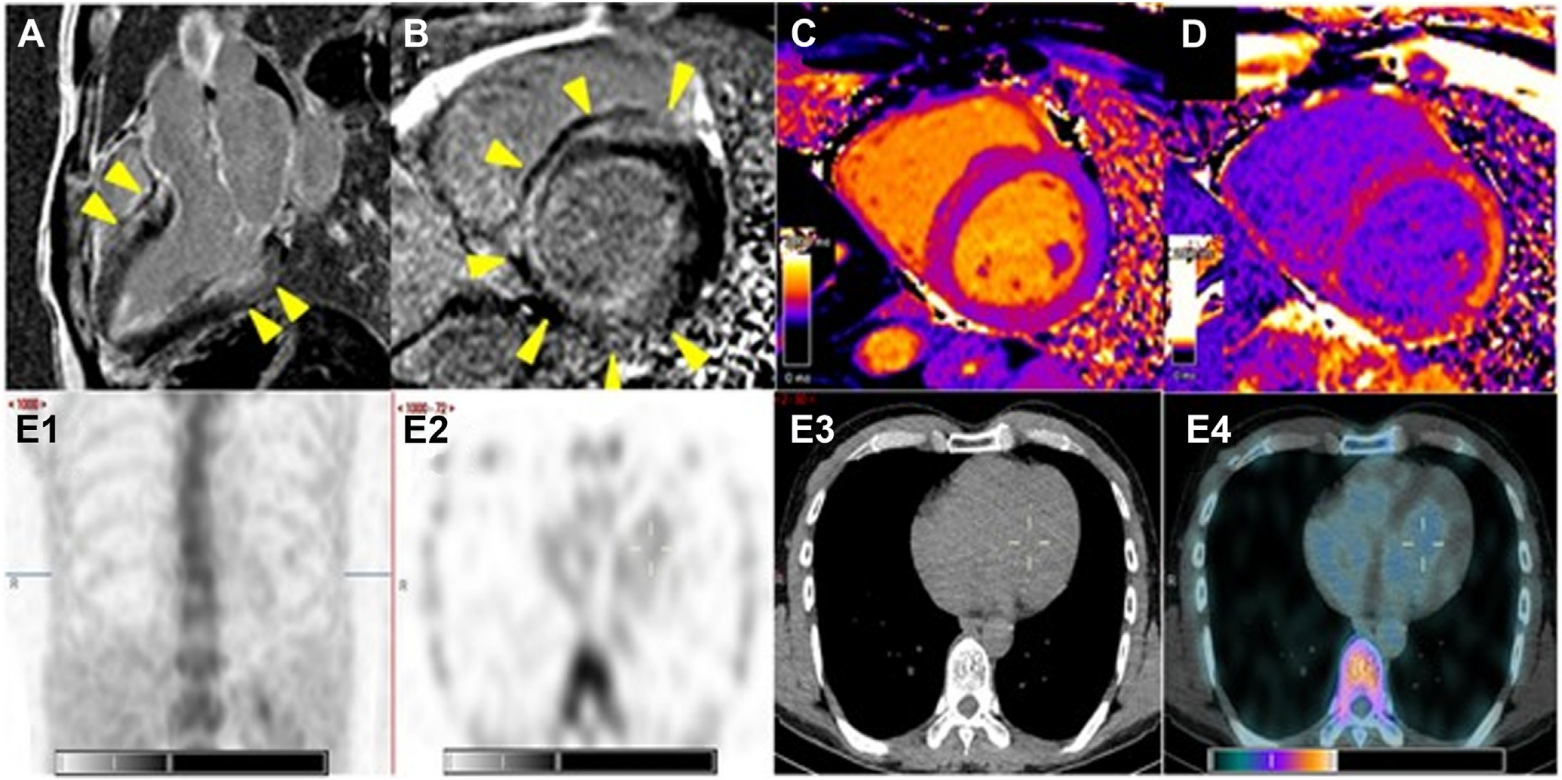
Postcontrast cardiac magnetic resonance with subendocardial to mid-wall late gadolinium enhancement of the basal myocardial segments in 3-chamber view (A) and basal short axis view (B). Precontrast T1-mapping (C) showed mildly increased T1 relaxation time in the basal segments, whereas the postcontrast T1 relaxation time was markedly reduced (D) allowing calculation of increased extracellular volume values between 37% and 40%. The ^99m^Tc-DPD scintigraphy showed no cardiac uptake, indicated as Perugini Grade 0 (E1-4). Trace uptake was noted at the bone level in E1. Imaging details: The cardiac imaging was performed using a Siemens Symbia Intevo 16 camera (Swedesboro, NJ) with a low-energy high-resolution (LEHR) collimator. The dose of Tc-99m-DPD was 8Mbq/kg with a scanning speed of 12 cm/min. Thorax static images (A/P) were acquired with 1000 kcts. SPECT/CT of the thorax used a 256 x 256 matrix, with 60 images per detector, each lasting 15 seconds in a noncircular orbit, continuous mode. Reconstructions were performed using FLASH 3D and XQUANT for quantification. The radiotracer injection was done at 10:13 AM, and image acquisition took place at 02:06 PM. SPECT/CT, single-photon emission computed tomography with a computed tomography scan.
